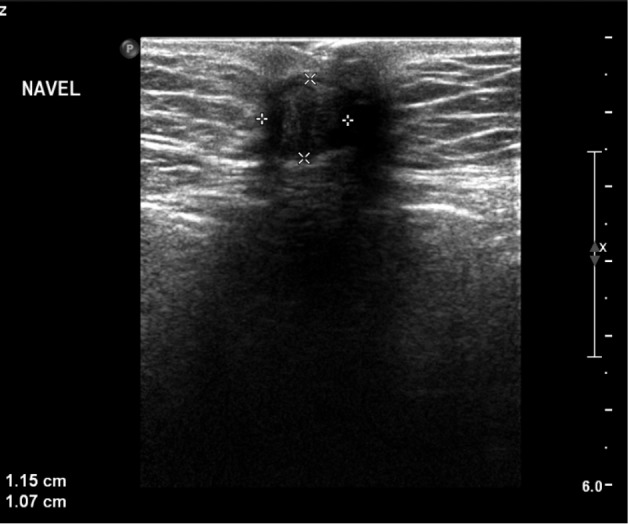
The abdominal ultrasound, showing a superficial nodule of 1.15 to 1.07cm in the umbilicus.
